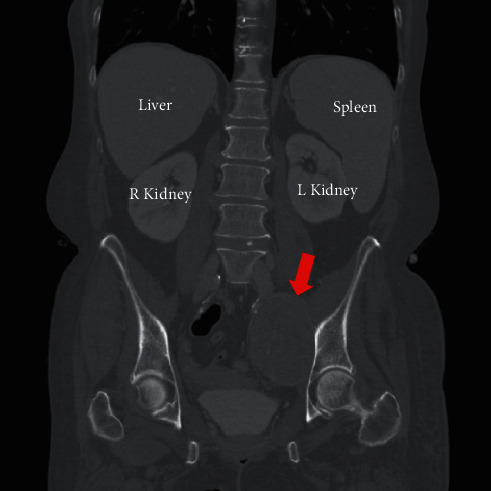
Computed tomography of the abdomen reveals 8.7 × 6.9 × 8.5 cm mass (red arrow) in the retroperitoneal space.
